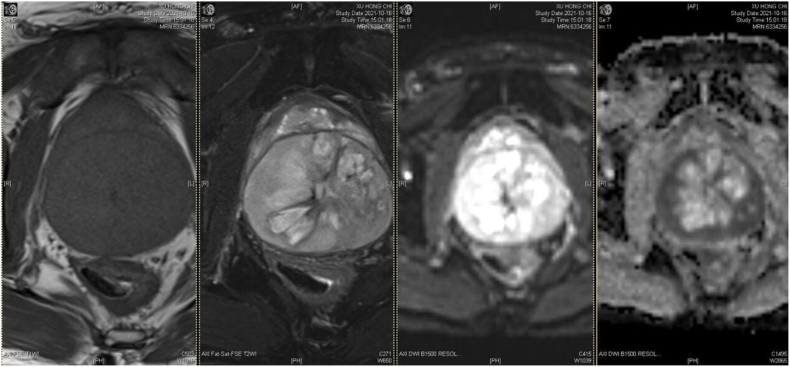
mpMRI multiparametric MRI, DWI diffusion weighted MRI, ADC Apparent diffusion coefficient.
